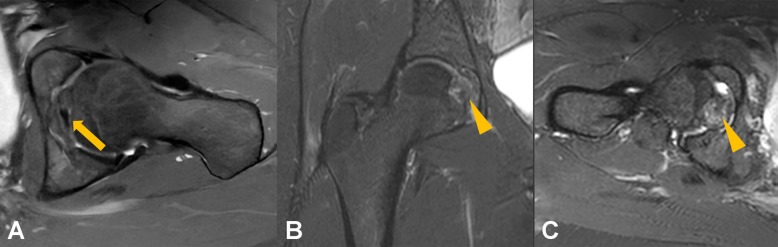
Representative coronal and transverse fat-suppressed T2-weighted images (A, B) of a 31-year-old woman without hip pain (A) and a 33-year-old woman presenting with atraumatic hip pain (B, C). Thickening of the ligamentum teres was observed in the asymptomatic control (arrow, A). The notable signal increase and thickening of the ligamentum teres in the patient with hip pain are marked by the arrowheads in (B, C)
